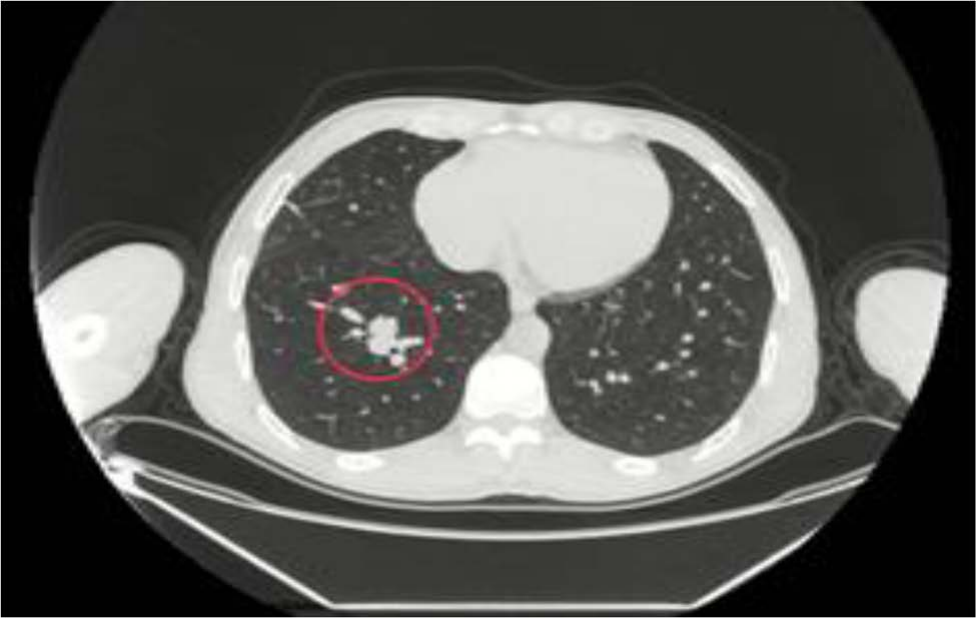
Chest CT scan, transverse view, demonstrating a spiculated mass in the right lower lobe of the lung (red circle). CT = computed tomography.
